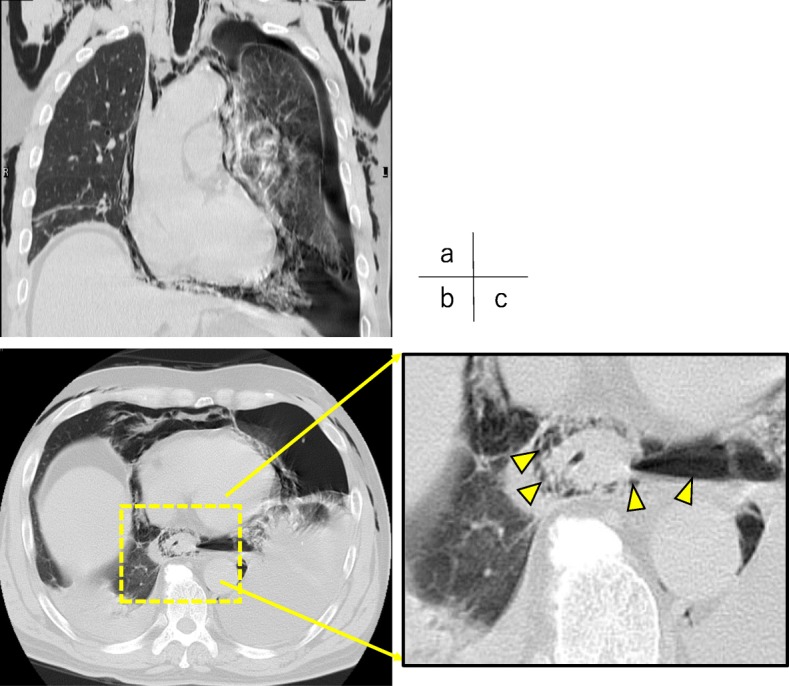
Preoperative chest CT findings. Chest CT revealed left tension pneumothorax (a) and pneumomediastinum around the lower esophagus (arrowheads) (a–c). a Transverse section. b Lower esophagus. c Enlarged view of the lower esophagus
